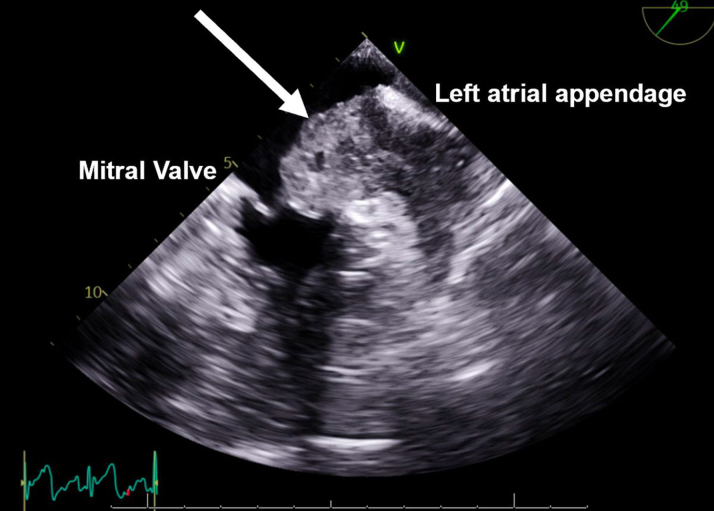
Transesophageal echocardiography Showing a Left Atrial Appendage Mass Without Crossing the Mitral Valve The arrow points to the left atrial appendage mass.
